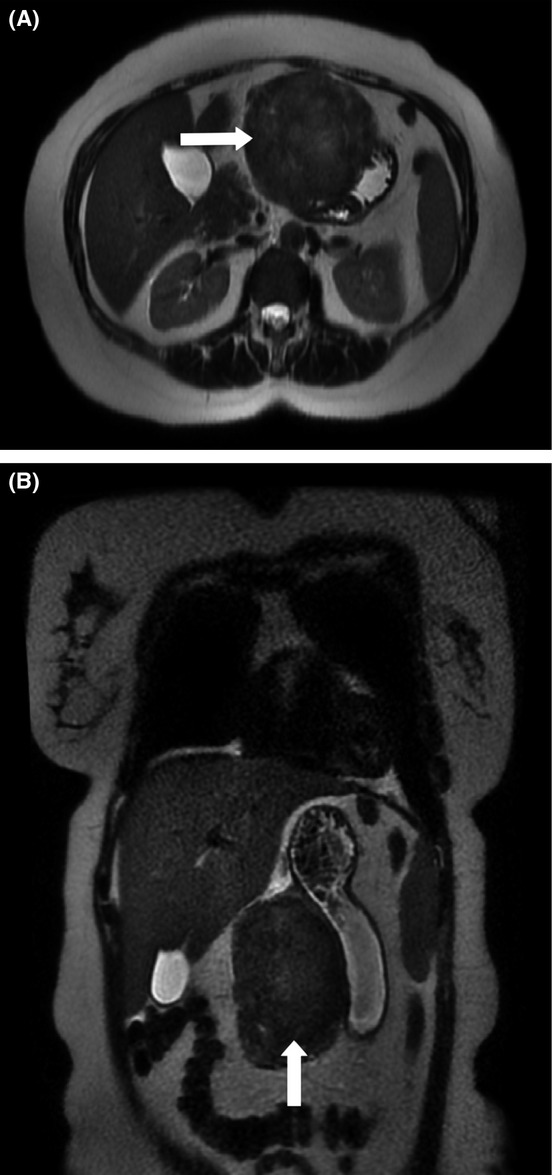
Abdominal MRI confirmed a large discretely marginated mass centered in the region of anterior stomach wall and the lesser curvature.
